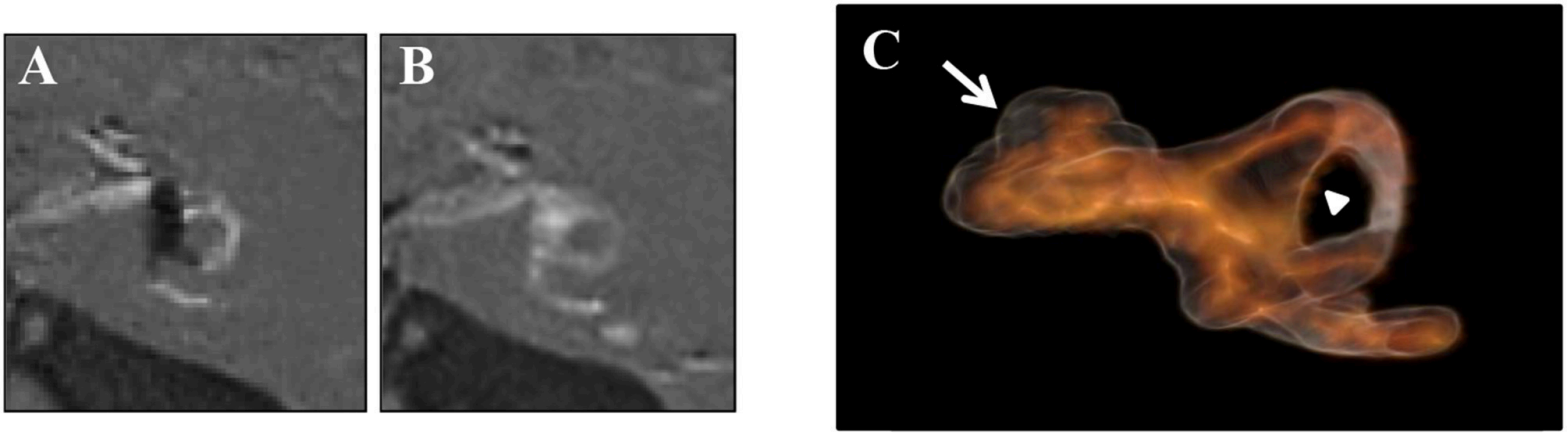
Changes in MRI images after ELSD. (A) Images of “PPI-PEI” before ELSD. (B) Images of “PPI-PEI” after ELSD. (C) Reconstructed 3D images of inner ear. There are non-enhanced areas with Gd, suggesting the existence of EH, in the cochlea, vestibule, and semicircular canals (SCCs) of the image obtained before ELSD (A). In contrast, non-enhanced areas in the vestibule and SCCs disappeared after ELSD (B). On reconstructed 3D image, light-browned area represents the perilymphatic space and the black area represents ELS (C). White arrow indicates ELS (=EH) in the cochlea and arrow head indicates ELS (=EH) in the vestibule.
